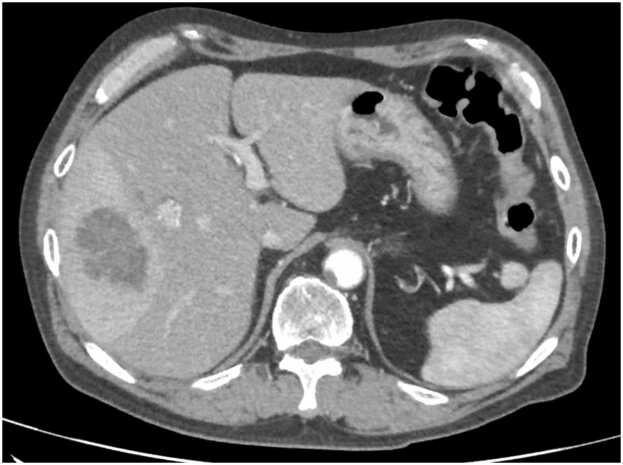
Contrast-enhanced computed tomography of the abdomen. Multiple lobulated low-attenuation areas are observed in the liver, with wedge-shaped areas of early enhancement surrounding them in the arterial phase.
